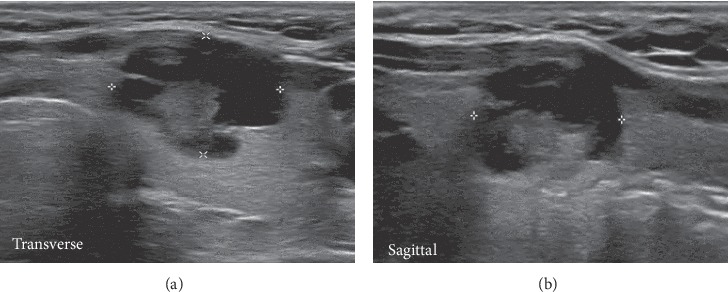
(a, b) Ultrasonograms (transverse and sagittal views), demonstrating a fairly oval-shaped solid isoechoic nodule with mixed solid and cystic components (1.38 × 1.14 × 1.36 cm) in the middle portion of the left thyroid lobe.
